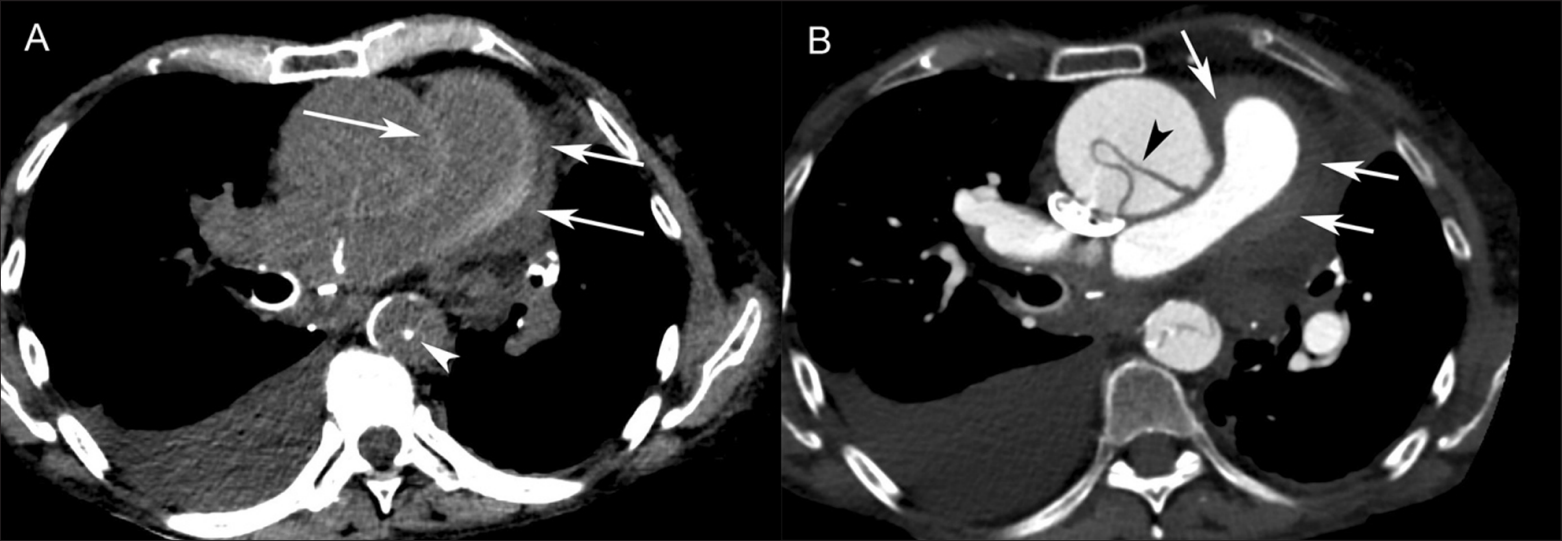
Patient 1. (A) Axial non-contrast-enhanced CT showed circumferential high attenuation along the walls of the pulmonary trunk (70 HU) and right pulmonary artery (arrows), consistent with an intramural hematoma. An ascending aortic aneurysm with displacement of atherosclerotic calcifications into the lumen of the descending aorta (arrowhead) was seen. Right pleural effusion was also visible. (B) Contrast-enhanced CT revealed Stanford type A aortic dissection. The intimal flap was seen in the ascending aorta (arrowhead) and descending aorta. The high attenuation area of the pulmonary arterial wall was non-contrast-enhancing (arrows), which was consistent with pulmonary artery intramural hematoma.
